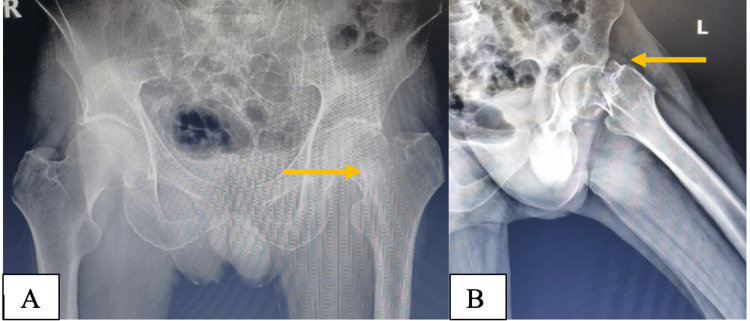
A- Anteroposterior pelvic radiograph. B- Lateral radiograph of the left hip. Both showing a displaced left hip fracture indicated by the yellow arrow.
